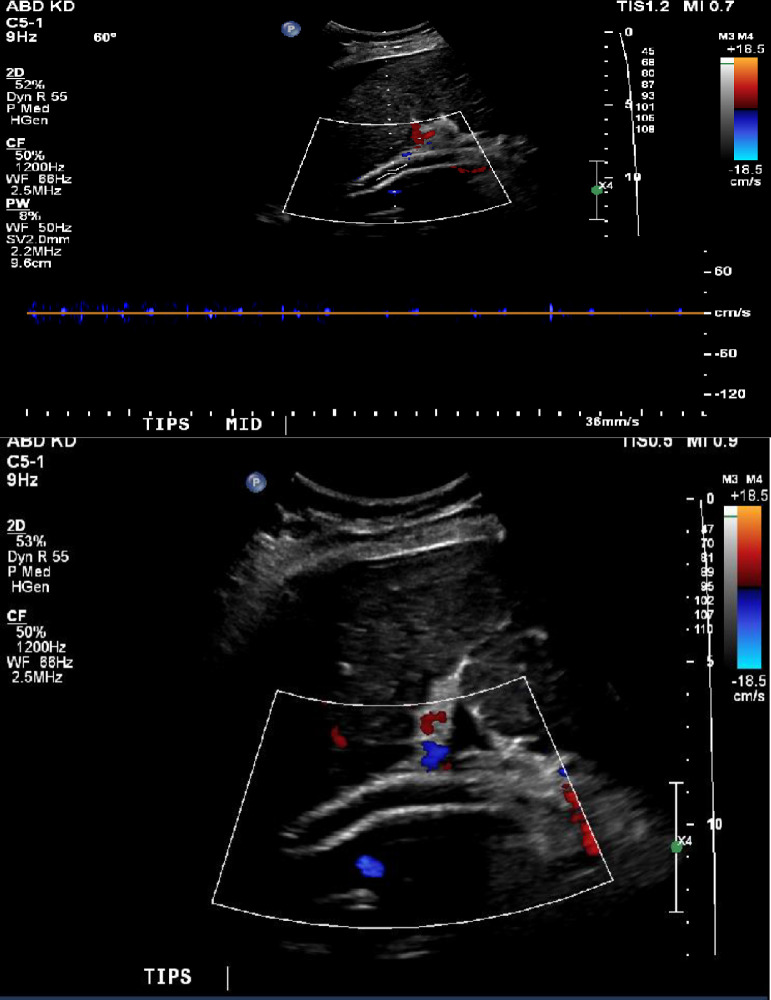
Duplex ultrasound images of the TIPS stent showing no blood flow within the TIPS shunt.
